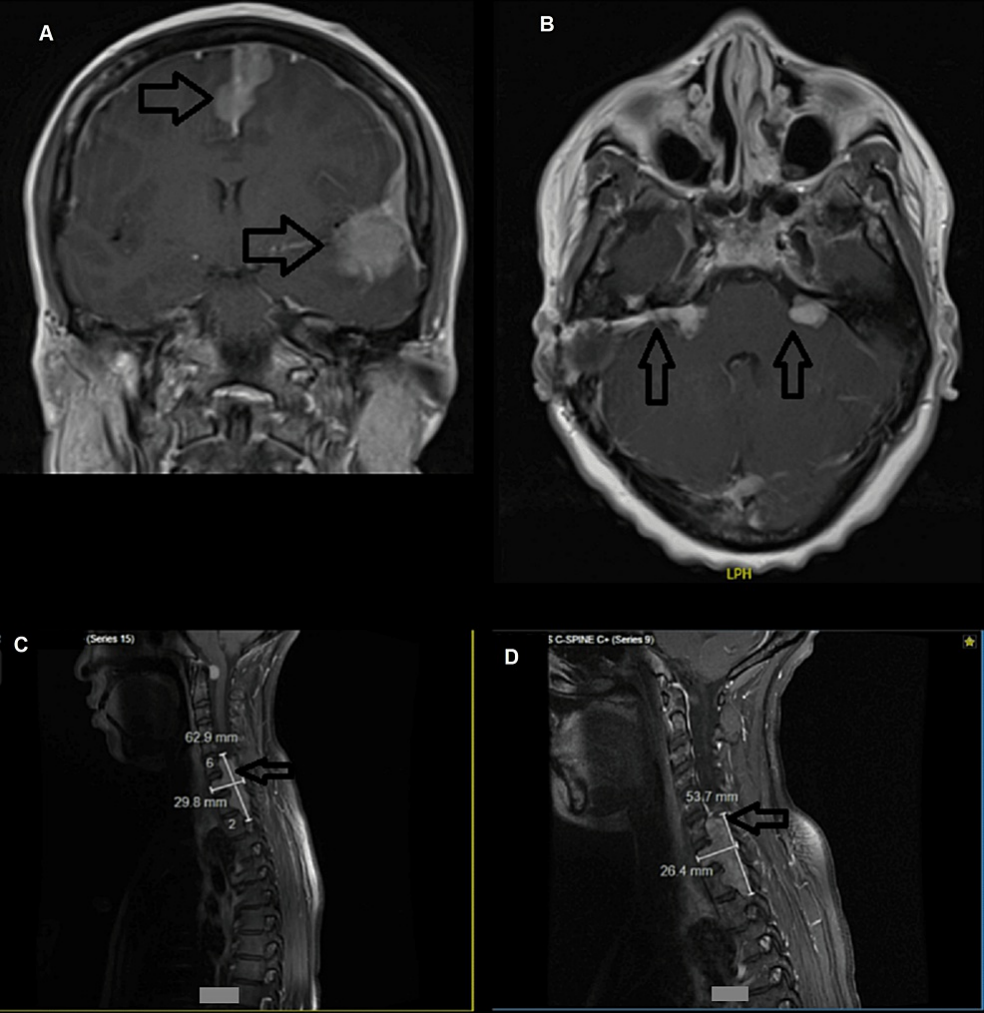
Gadolinium-enhanced T1 weighted MRI of the brain and spinal cord (A) Coronal sequence demonstrating parasagittal and left temporal homogenously enhancing lesions suggesting meningioma (arrows); (B) Axial sequence with characteristic bilateral vestibular schwannoma (arrows); (C) Sagittal sequence depicting a cervicothoracic extramedullary enhancing lesion prior to treatment with bevacizumab with dimensions of 62.9 x 29.8 mm (arrows); (D)Sagittal sequence depicting the same lesion seven months after treatment with bevacizumab with dimensions of 53.7 x 26.4 mm (arrows)
